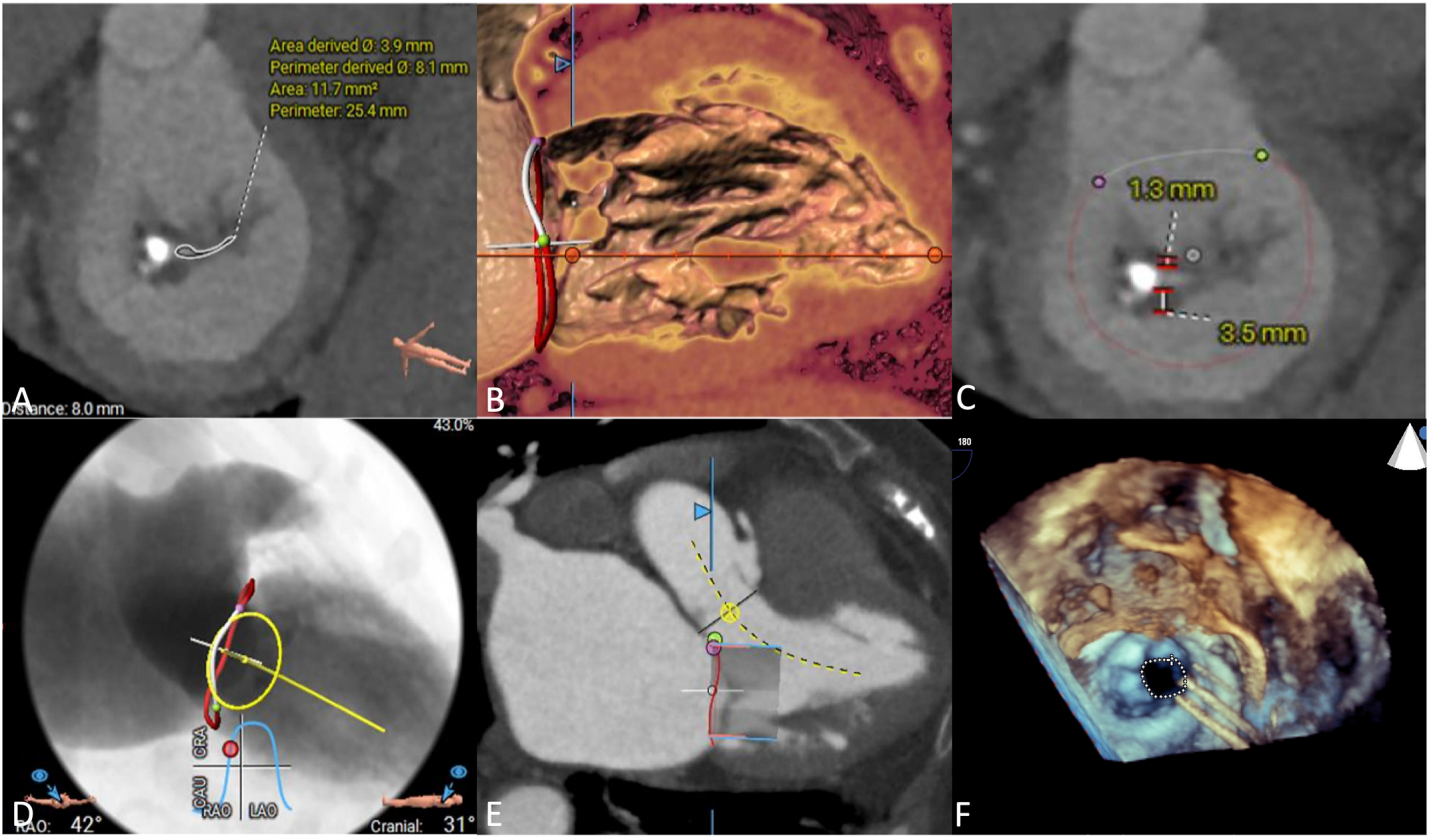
Preprocedural imaging assessments. (A) Mitral annulus measurement, the annular area was 11.7 mm^2^. (B) Subvalvular apparatus evaluation, chordae tendinae was shown thickened. (C) Leaflets of mitral valve evaluation. (D) Implanted projection angle determination. (E) Neo-left ventricular outflow tract prediction. (F) Rheumatic mitral stenosis was displayed in preprocedural echocardiography.
